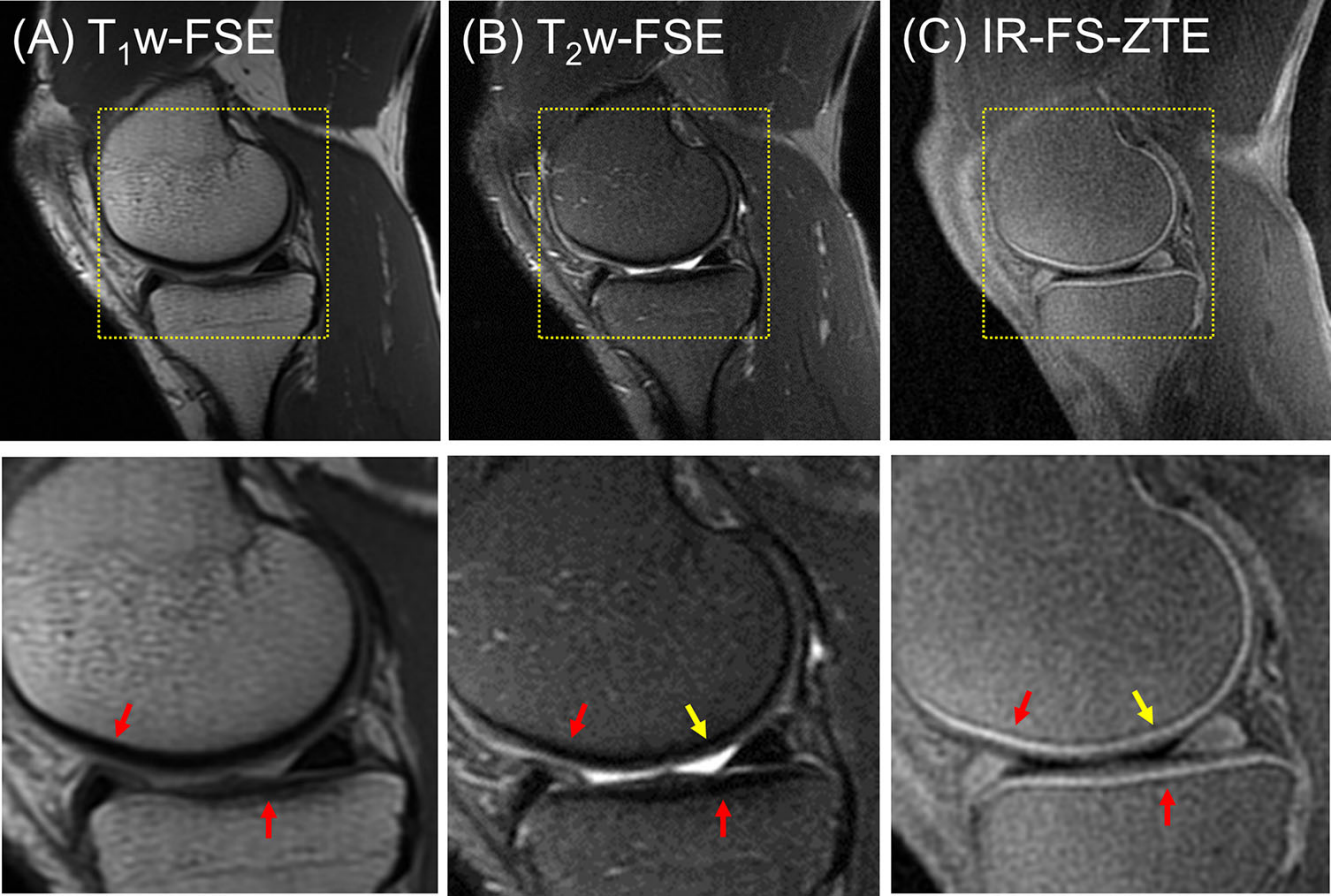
A healthy volunteer (35-year-old male). (A) T_1_w-FSE, (B) T_2_w-FSE, and (C) IR-FS-ZTE images (top) and the corresponding zoomed-in images (bottom). The short T_2_ signal from the OCJ region is resolved with high contrast in IR-FS-ZTE imaging (C), while the signal is not captured at all by the conventional clinical MR imaging sequences (A, B), as indicated by red arrows. The joint fluid with long T_1_ and T_2_ appears bright in T_2_-FSE imaging, but dark in IR-FS-ZTE imaging (yellow arrow).
